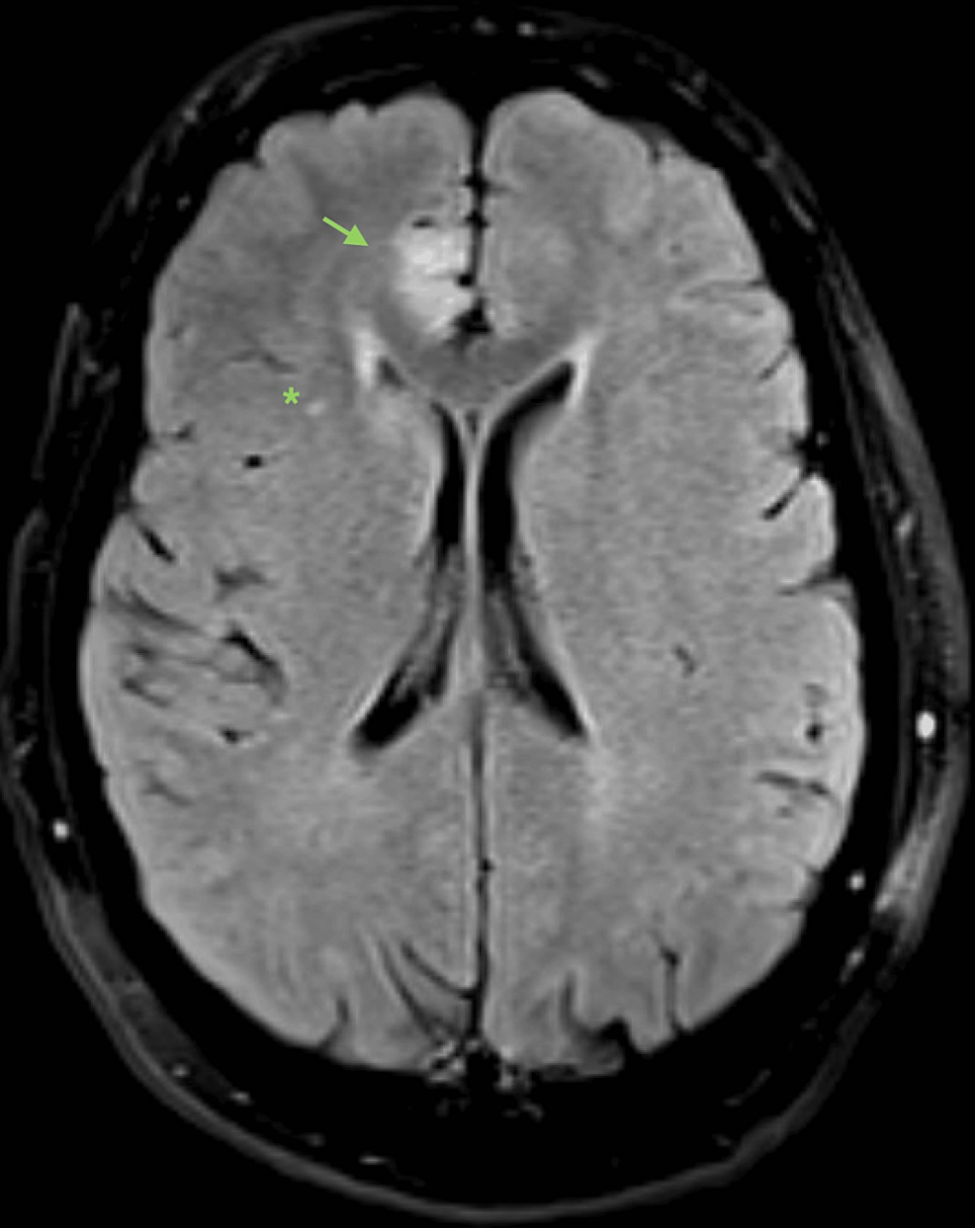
Fluid-attenuated inversion recovery magnetic resonance imaging (FLAIR MRI) from admission day 2, axial section, showing a right mesiofrontal hyperintense focus (arrow) and a right subcortical punctate hyperintense focus (*).
